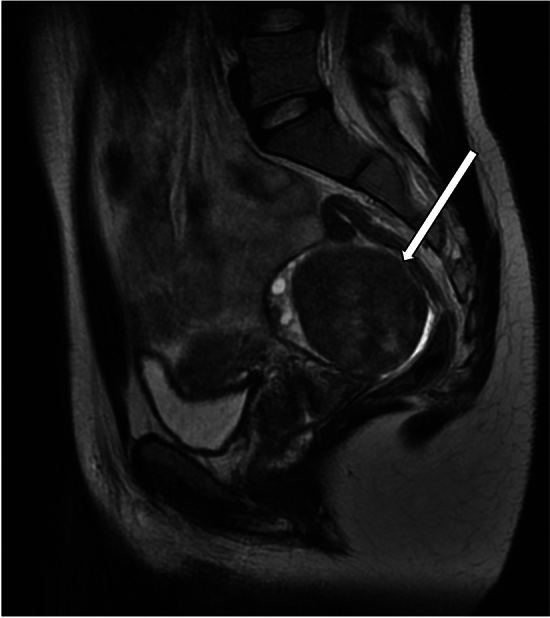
Pelvic MRI in the same patient shows a solid left ovarian lesion with low to intermediate T2 signal intensity (arrow), as well as mild uniform enhancement, consistent with fibrous tumor such as fibrothecoma. The patient underwent left salpingo-oophorectomy, with final pathology consistent with ovarian fibrothecoma
